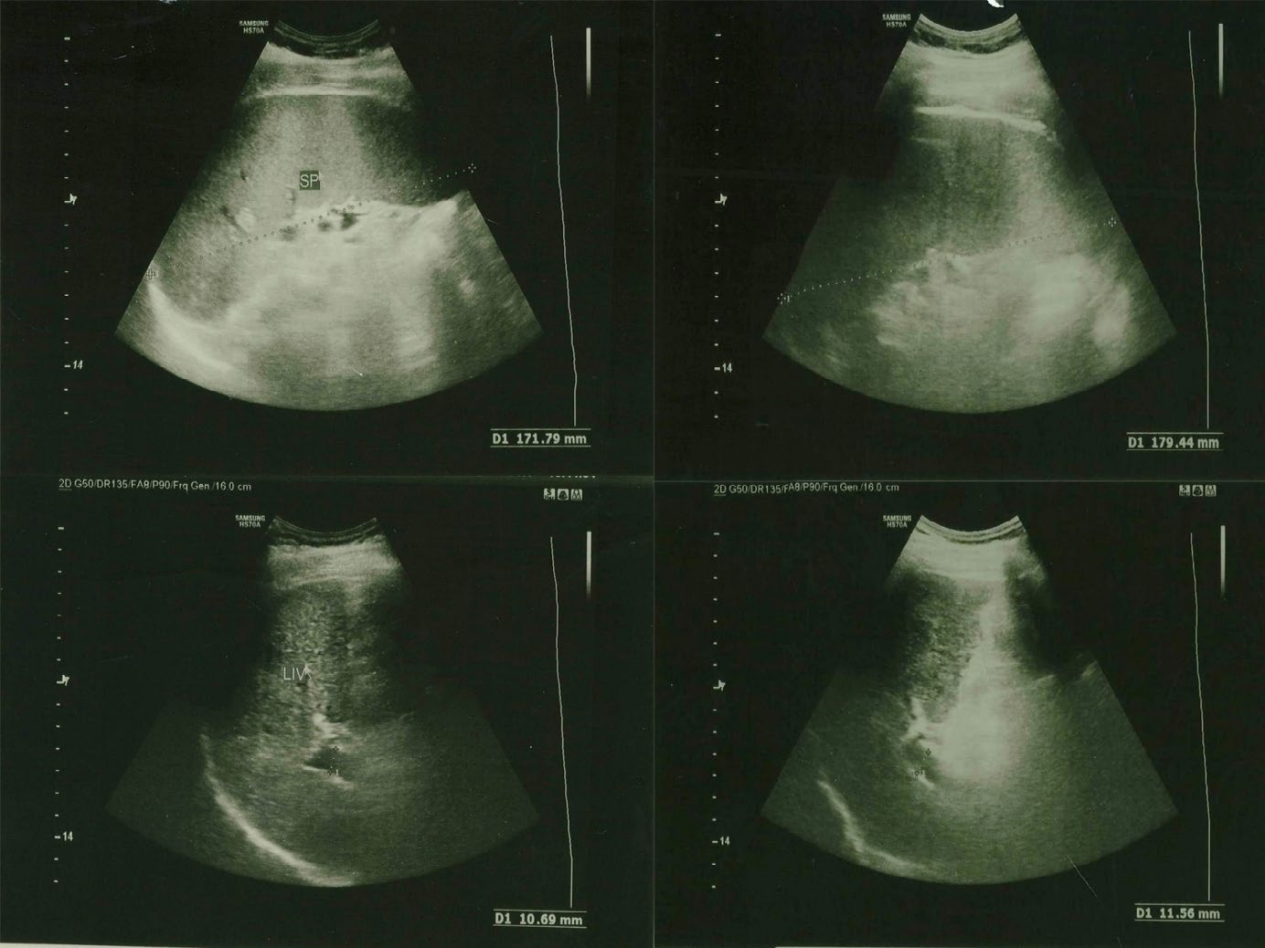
Ultrasonography of liver and spleen. Splenomegaly was detected
